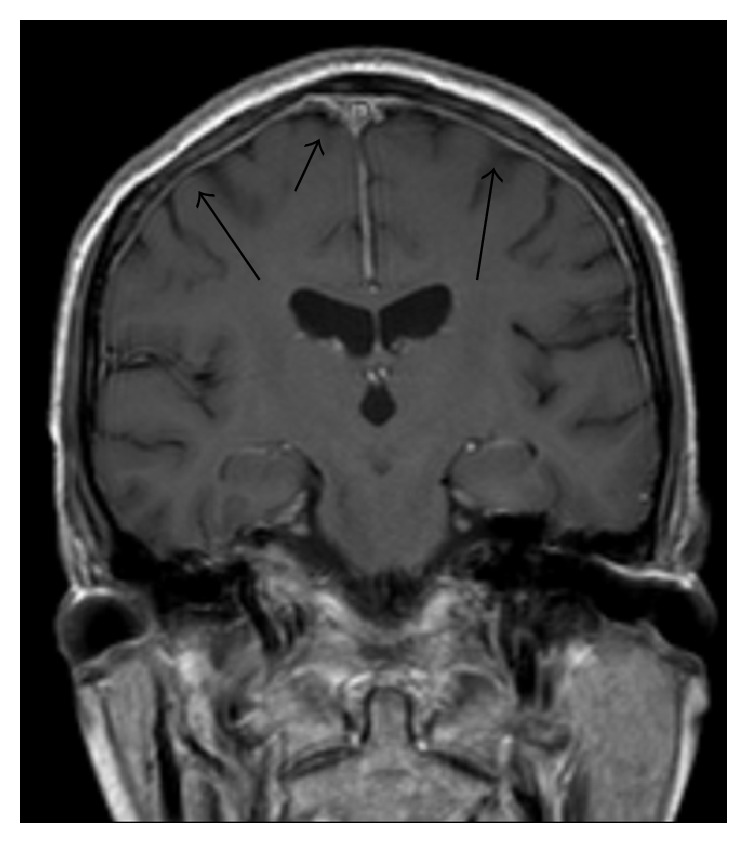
MRI brain coronal view shows diffuse meningeal enhancement.
